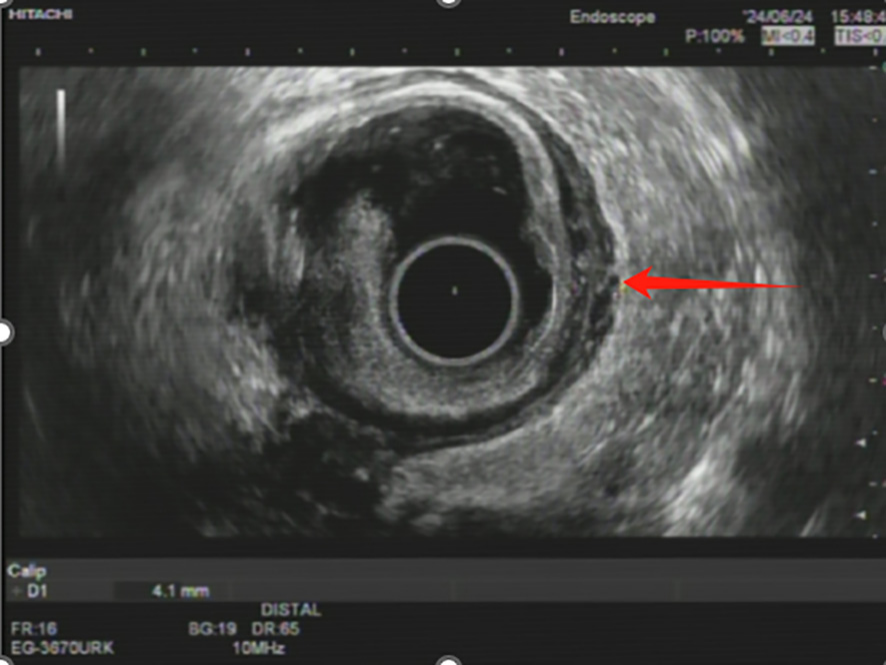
Endoscopic ultrasonography image showing post-treatment changes in rectal wall. The endoscopic ultrasonography (EUS) image demonstrates restoration of the rectal mucosa (red arrow) following multimodal therapy. The muscularis propria shows a layered structure with slight thickening (4.1 mm), consistent with treatment response.
